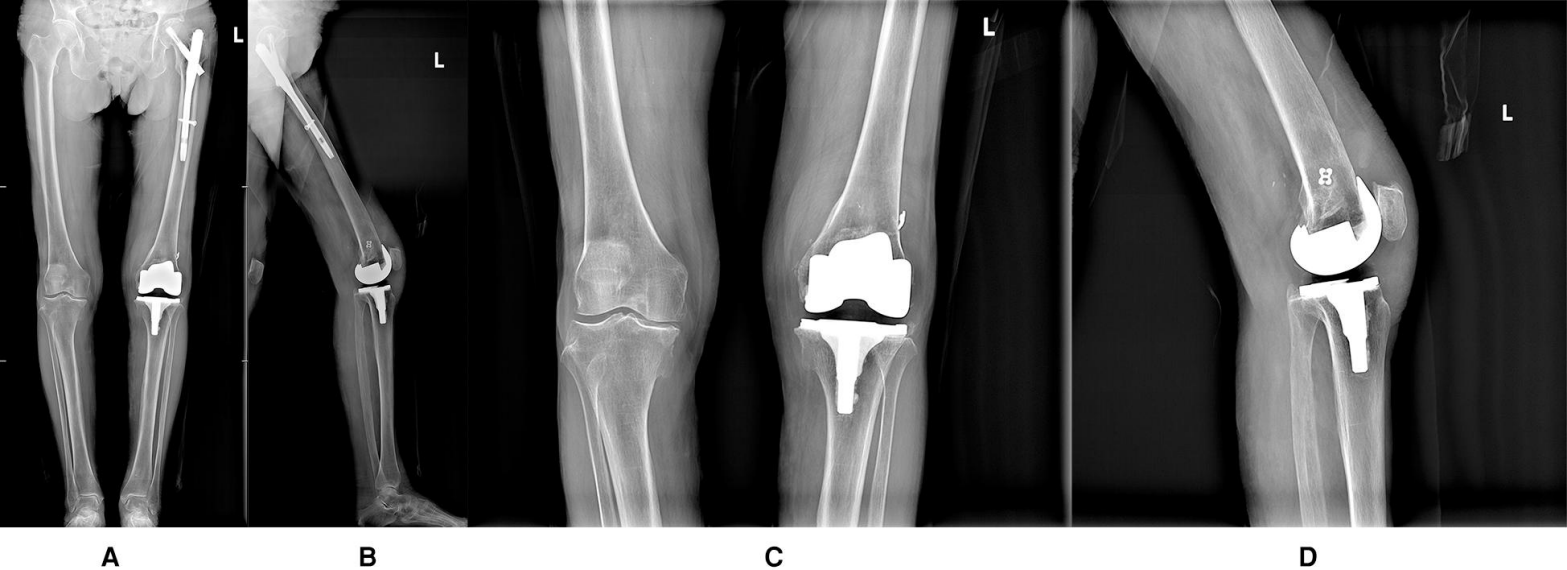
(A) Full-length frontal radiograph of the lower limb (The 7-month postoperative follow-up radiograph); (B) Full-length lateral radiograph of the lower limb (The 7-month postoperative follow-up radiograph); (C) Frontal radiograph of left knee joint (The 7-month postoperative follow-up radiograph); (D) Lateral radiograph of left knee joint (The 7-month postoperative follow-up radiograph).
